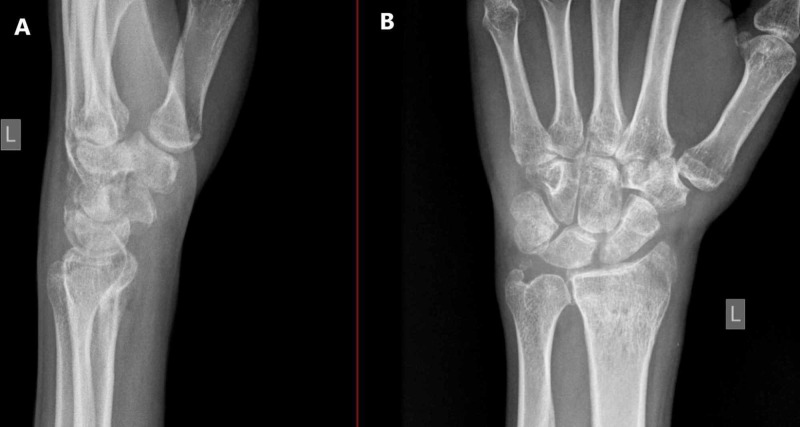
X-ray of the left wrist post wires removal surgery. A) Lateral view; B) anteroposterior (AP) view
